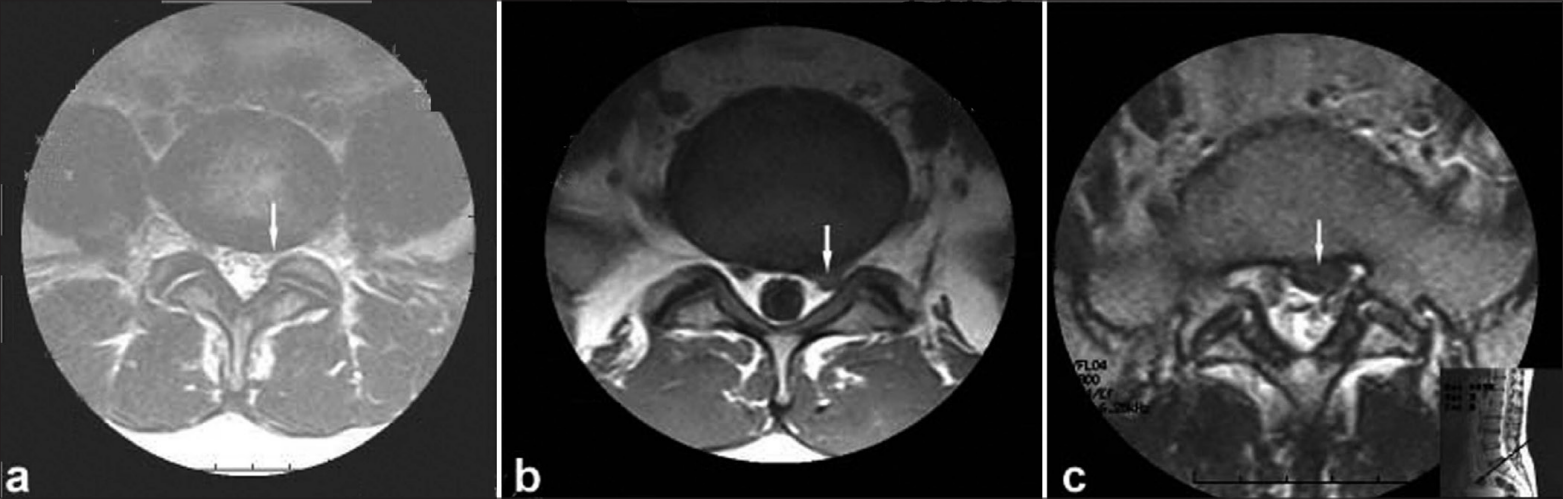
Axial section on MRI depicting classification of disc herniation. (a) Circumferential symmetrical disc extension beyond the interspace (Disc Bulge) (b) Focal or asymmetrical disc extension beyond the interspace with base against the parent disc broader than any other diameter of the protrusion (Disc protrusion) (c) Focal obvious disc extension beyond the interspace with base against the parent disc narrower than the diameter of the extruding material itself or no connection to parent disc (Disc extrusion)
